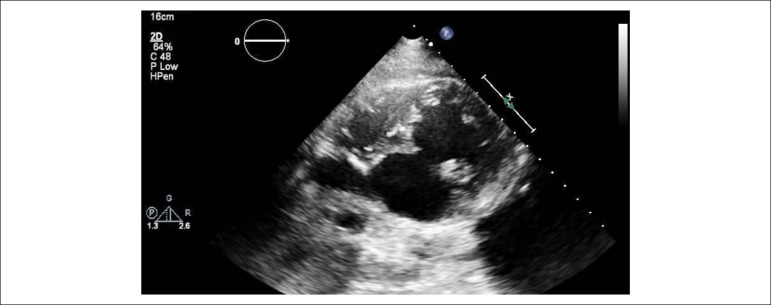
Transthoracic echocardiogram - modified subcostal window. Link: http://publicacoes.cardiol.br/portal/abc/portugues/2020/v11402/dor-abdominal-umaapresentacao-incomum-de-ruptura-miocardica.asp
